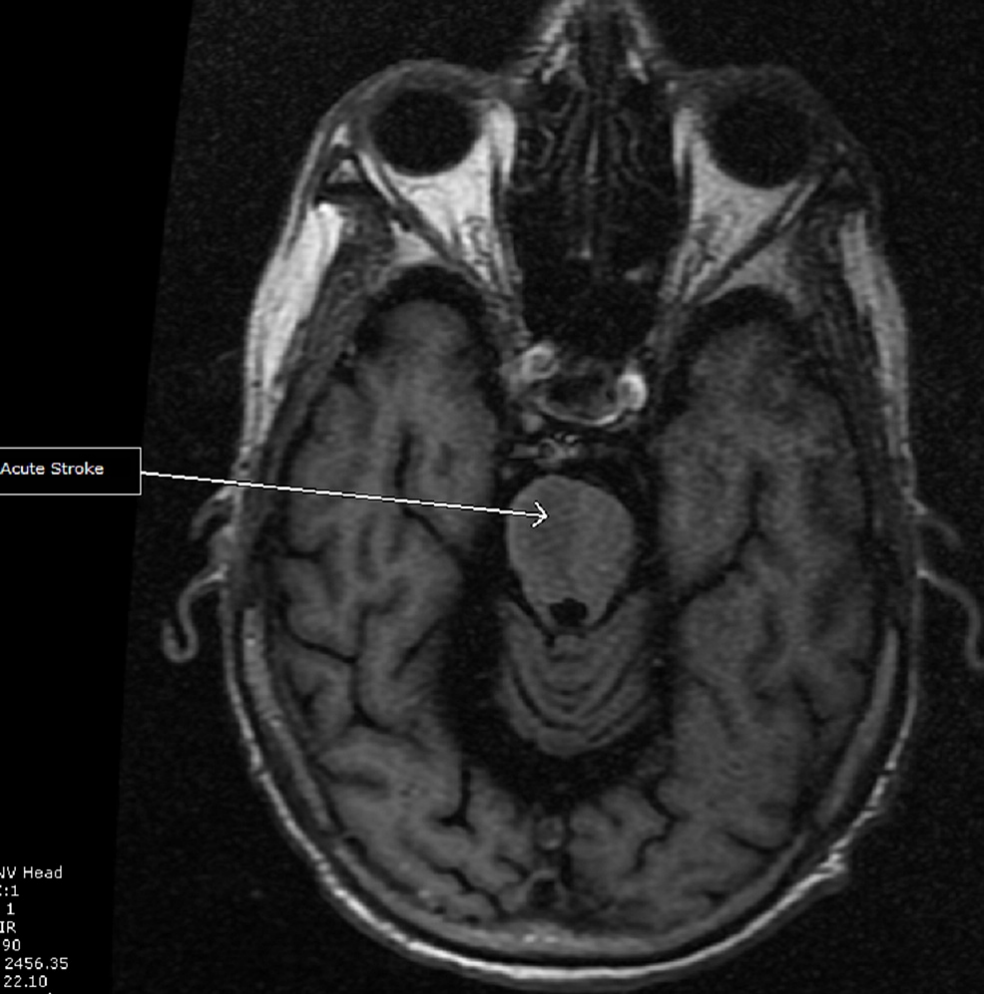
MRI T1 FLAIR with acute pontine stroke.
